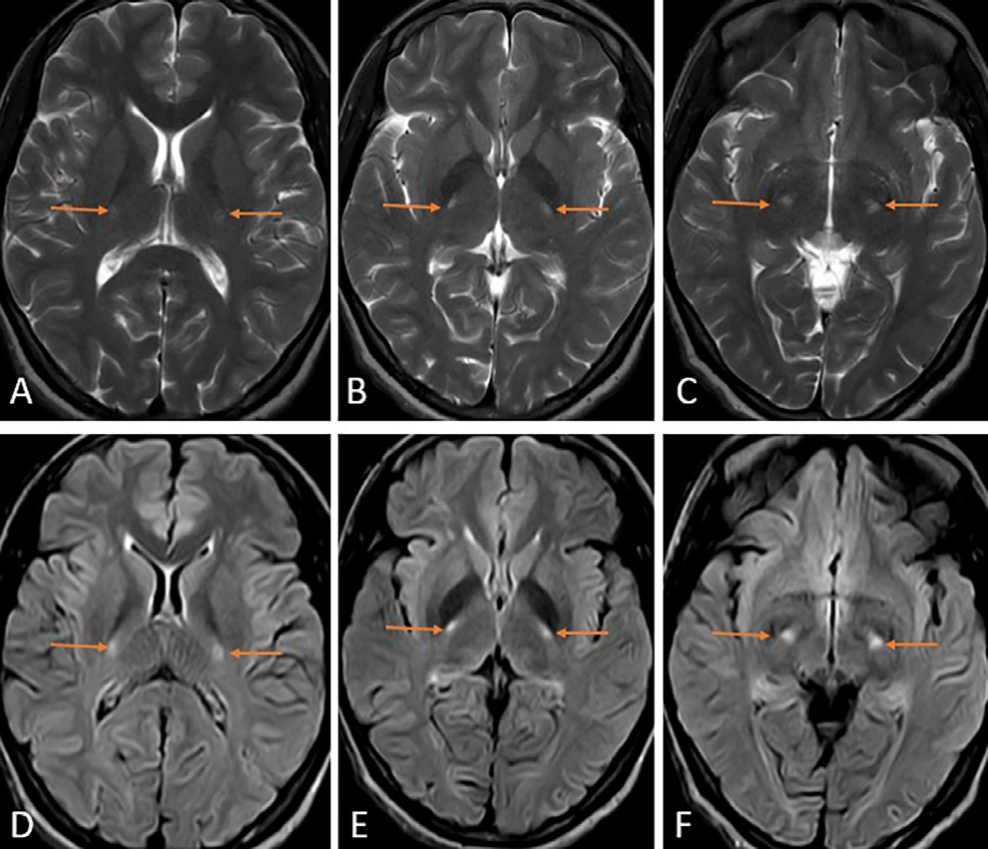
Magnetic Resonance Imaging of the brain axial sections T2 weighted imaging (A, B, C), fluid attenuation recovery sequence (D), (E), and (F) showing hyperintense signal involving bilateral corticospinal tracts, bilateral internal capsule, extending superiorly up to the motor cortex and inferiorly up to the cerebral peduncles of the midbrain (orange arrows) suggesting amyotrophic lateral sclerosis.
